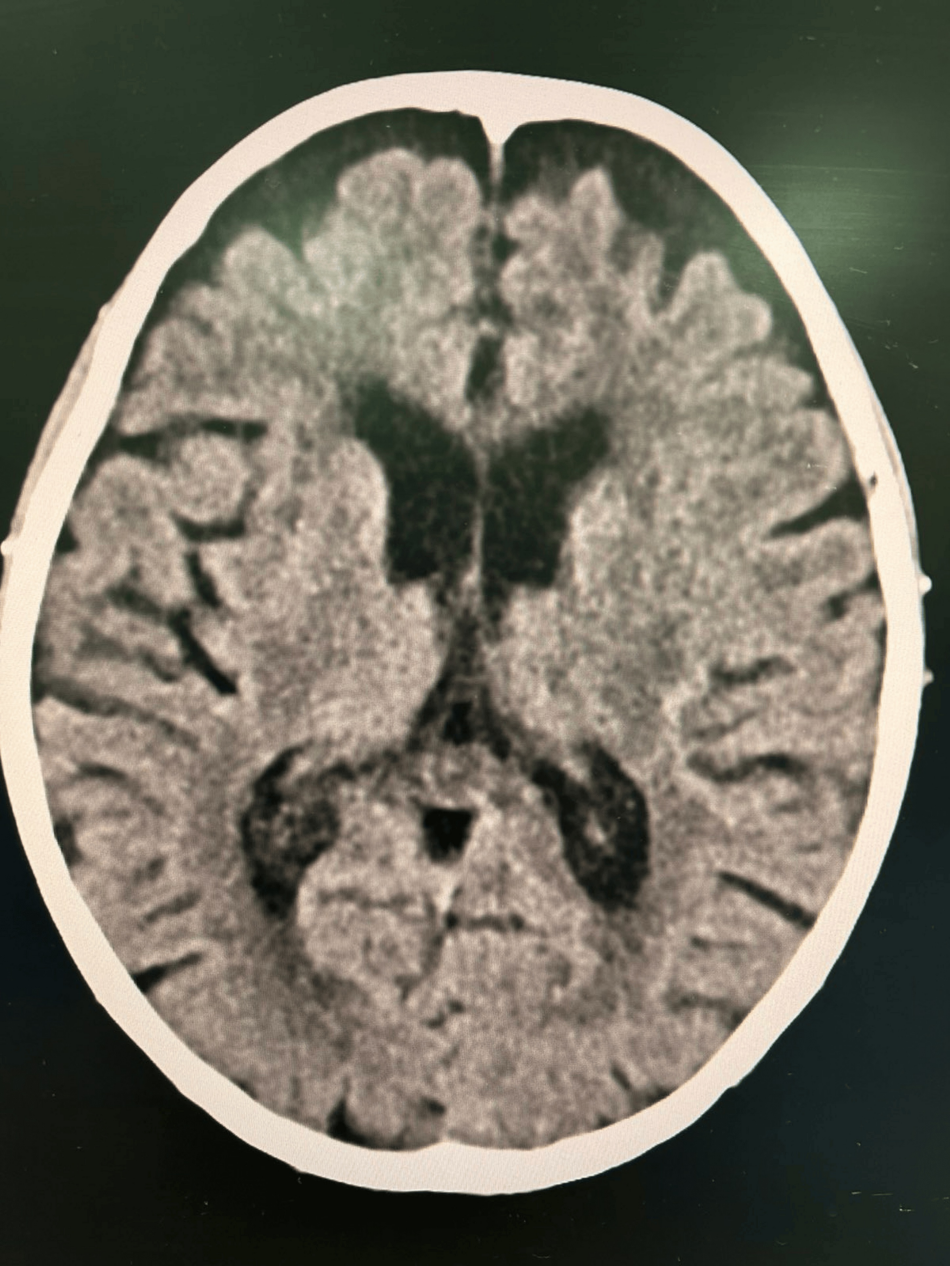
Axial view of the brain CT scan An unremarkable brain CT scan with evidence of age-related cerebral volume loss. CT: computed tomography
